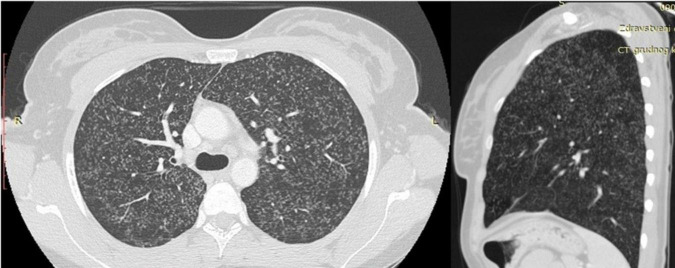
Computerized tomography (CT) of the chest with visible multiple micronodular changes in the lungs.
